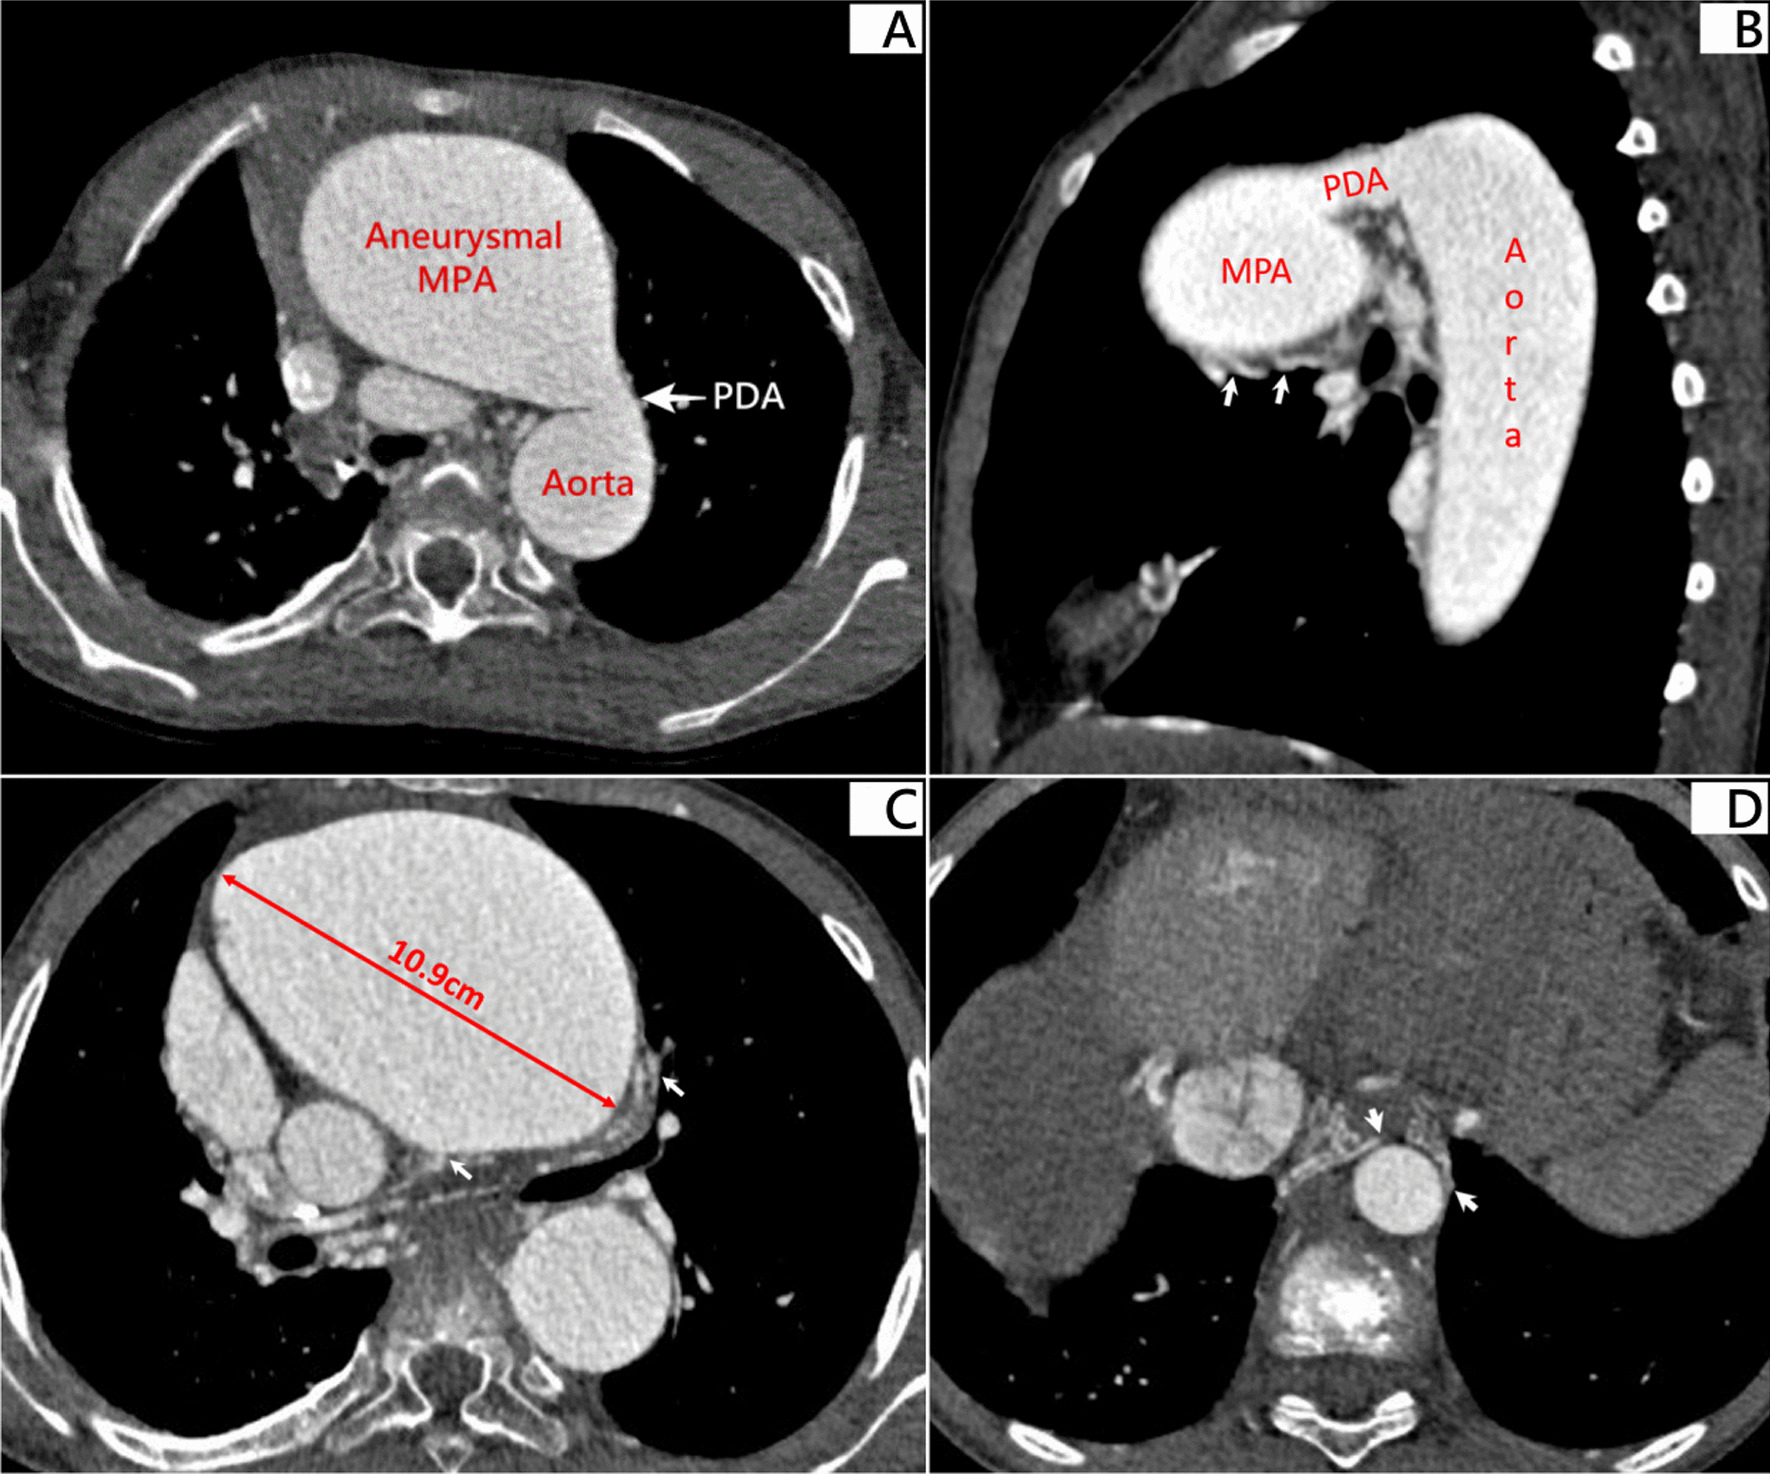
Contrast thoracic computed tomography shows the patient’s cardiothoracic anatomy before lung transplantation. A Patent ductus arteriosus (approximately 1.1 cm in diameter) identified between the aneurysmal main pulmonary artery and the descending aorta. B Axial view of thoracic computed tomography reveals patent ductus arteriosus between the main pulmonary artery and the descending aorta. Arrows indicate several collateral branches of bilateral pulmonary arteries (approximately 4–5 mm in diameter). C Maximum diameter of the aneurysmal main pulmonary trunk is 10.9 cm. The Arrows indicate several collateral branches of bilateral pulmonary arteries (approximately 4–5 mm in diameter). D Arrows indicate bilateral major aortopulmonary collateral arteries (MAPCAs) from the descending aorta (approximately 2–4 mm in diameter)
